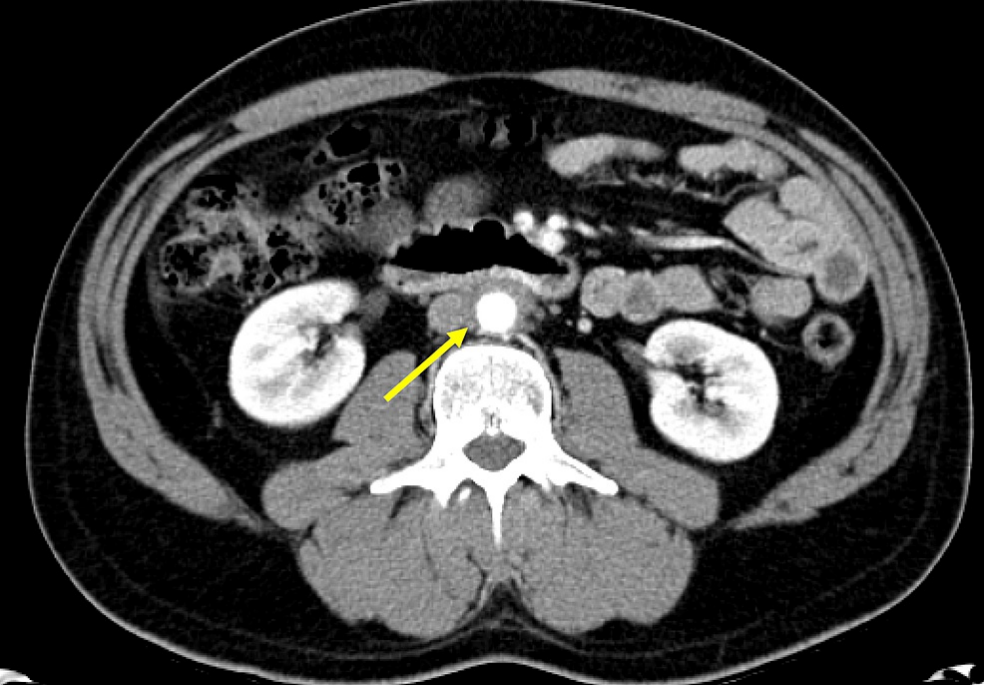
CT three months after initiation of treatment with prednisone demonstrating treatment effect There is a marked improvement in the circumferential soft tissue thickening (arrow) around the infrarenal abdominal aorta. This circumferential soft tissue measures up to 5 mm in thickness (decreased from 1 cm on prior CT). Again, there is no ureteral retraction or hydronephrosis.
